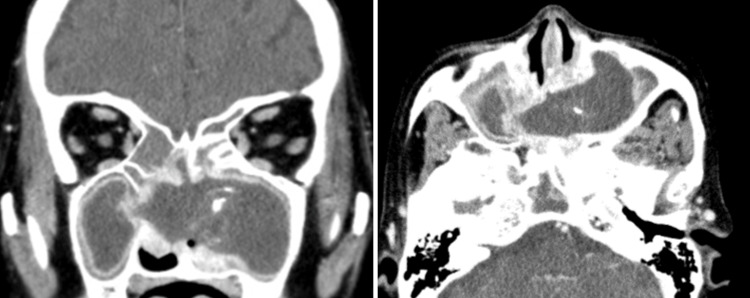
Contrast-enhanced sinus CT images Coronal and axial images show a mass lesion with enhanced margins and central calcification in the left maxillary sinus, extending to the contralateral maxillary sinus through the nasal septum. CT: computed tomography
